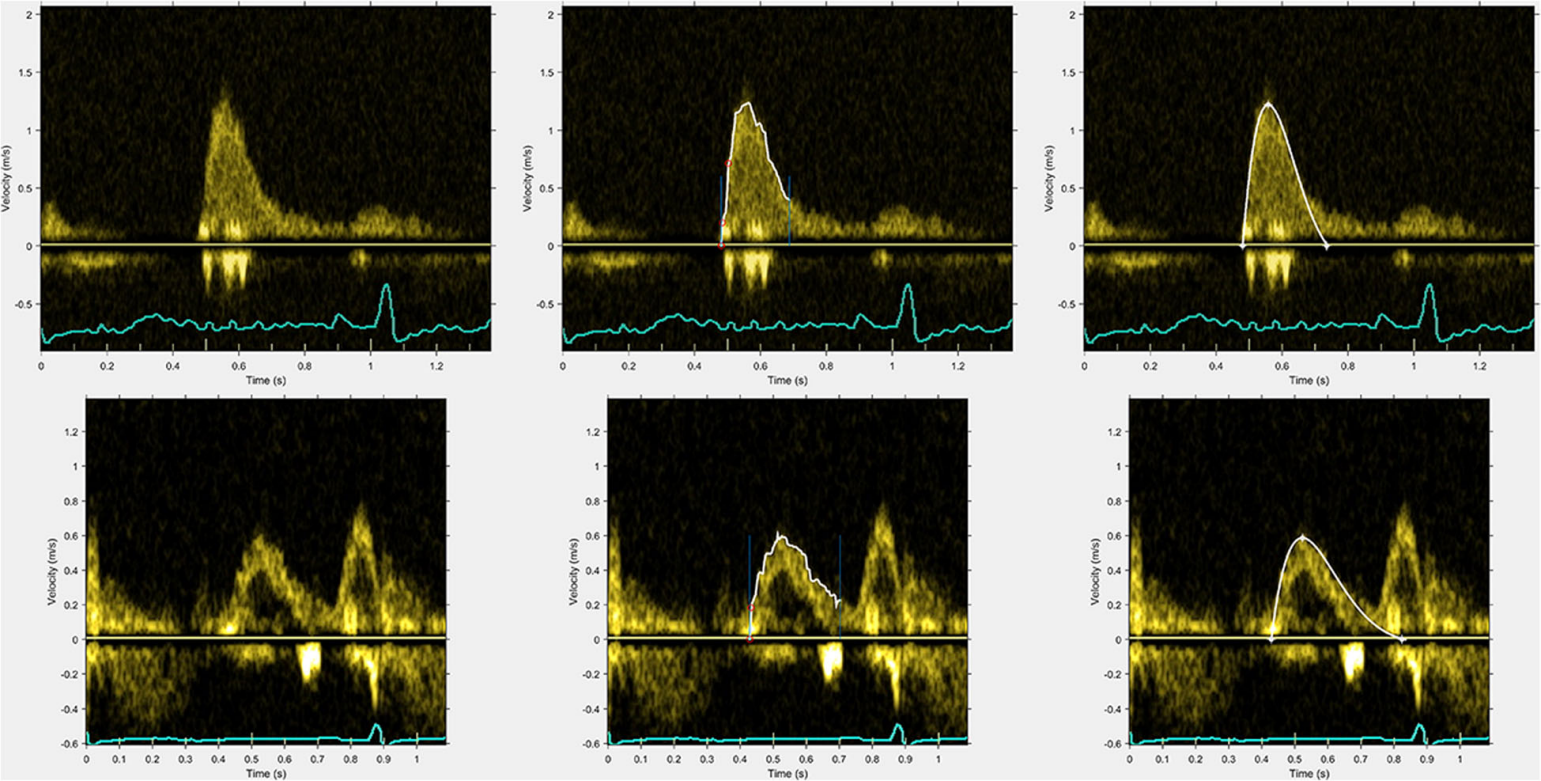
Representative examples of Doppler velocity profile edge detection and the resulting fitted *curves* for two different patients (*top* and *bottom*, respectively). *Left* panels: original Doppler images. *Mid* panels: detected edges of the velocity profiles in *white*. *Right* panels: fitted *curves* (*white*). PDF parameters for the *top row*: *c* 17.3 g/s, *k* 135 g/s^2^
